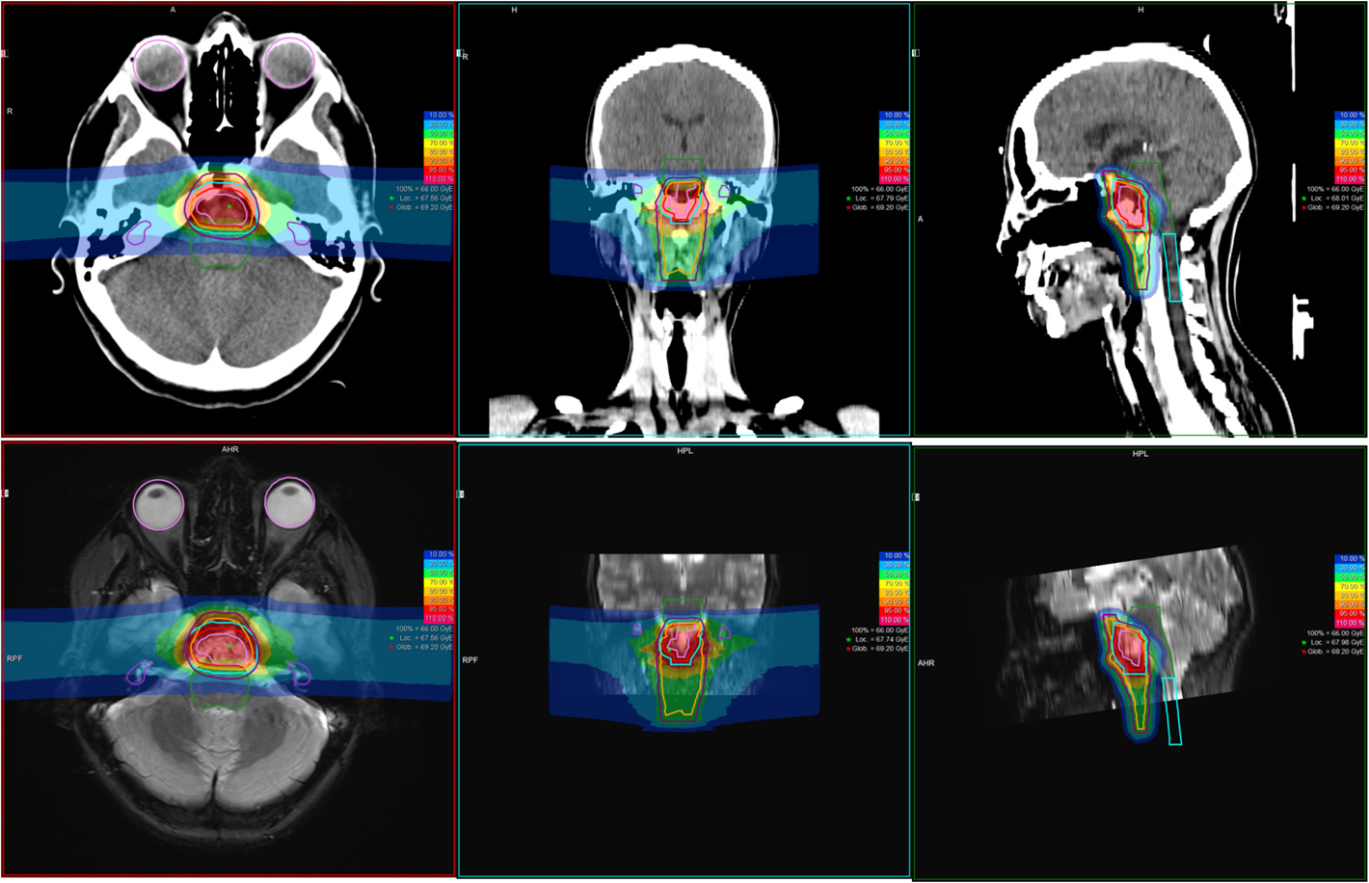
Clinical case of a clival chordoma treated with CIRT; Primary + Boost plan 66Gy (EQD) in 22 Fx. Top panel: CT scan; Bottom panel: T2w MRI (courtesy Dr. Uhl, HIT)
